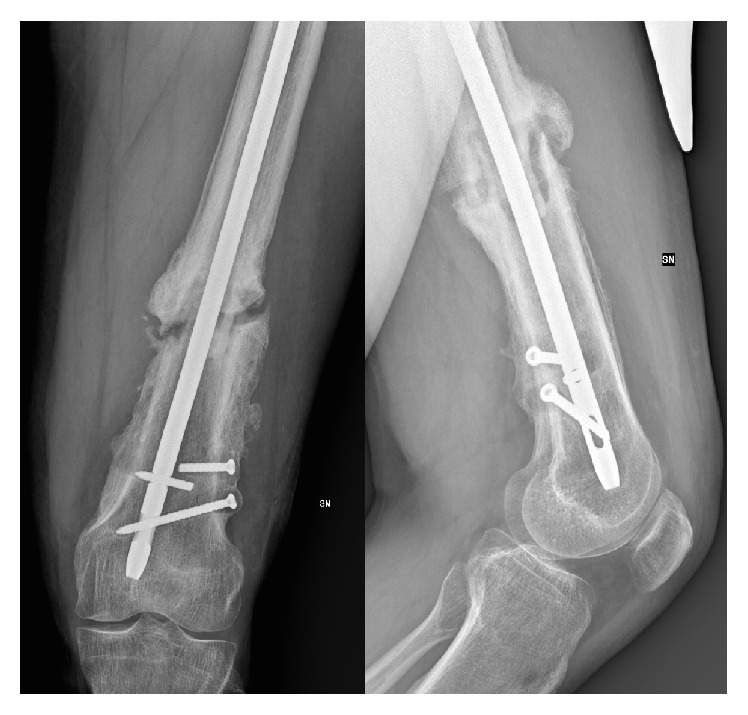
Preoperative radiographs showing an unconsolidated distal femoral shaft fracture with the rupture of one of the distal screws.
